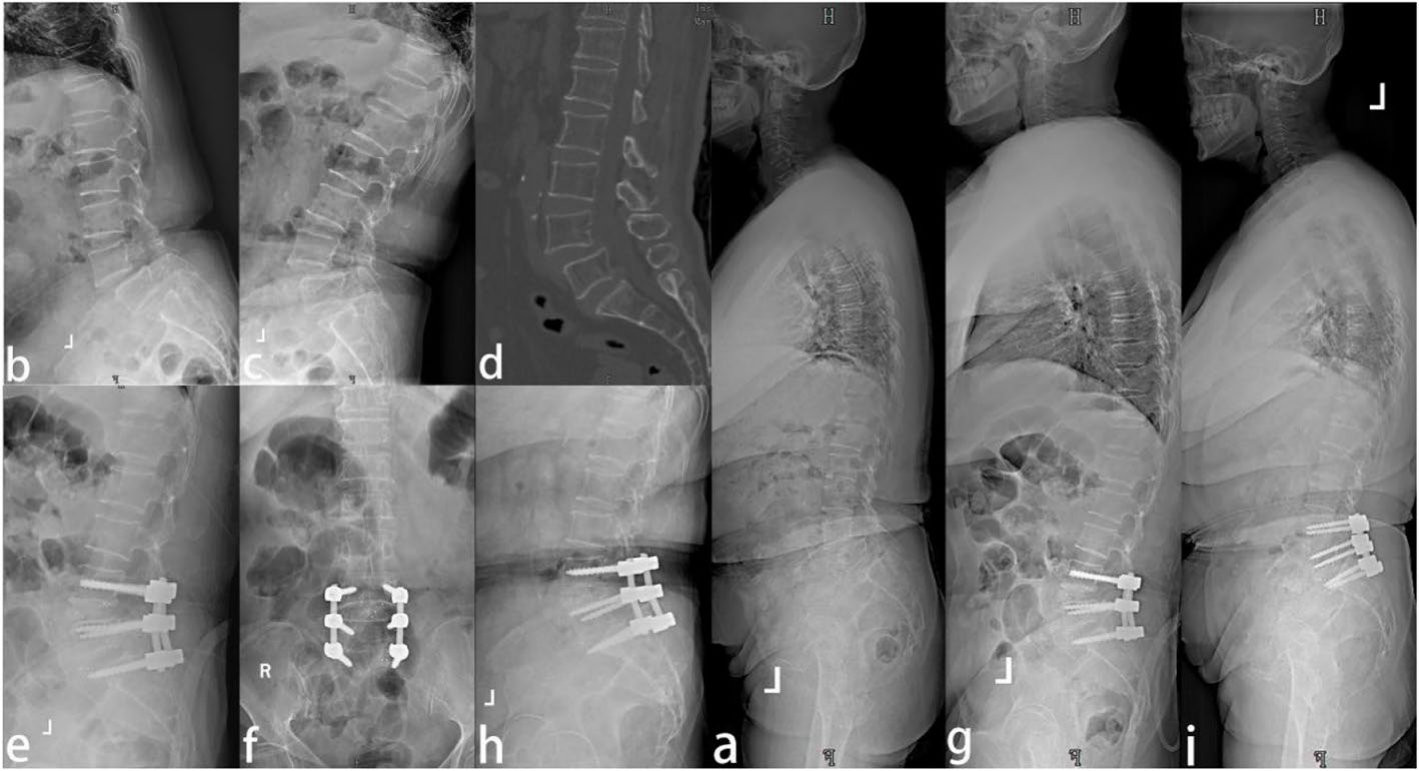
A 53-year-old female patient with L4/L5 two-level lumbar spondylolisthesis a, b, c refers to the preoperative lumbar lateral and whole spine lateral X-ray; d refers to the lumbar CT sagittal reconstruction, showing the anterior slippage of the vertebral body; e, f, g refers to the lumbar lateral and whole spine lateral X-ray after two-level unilateral TLIF, which shows that the sagittal imbalance is significantly improved; h, i refers to the satisfactory internal fixation position during the follow-up at 12 months after operation, and no loss of sagittal correction is observed
